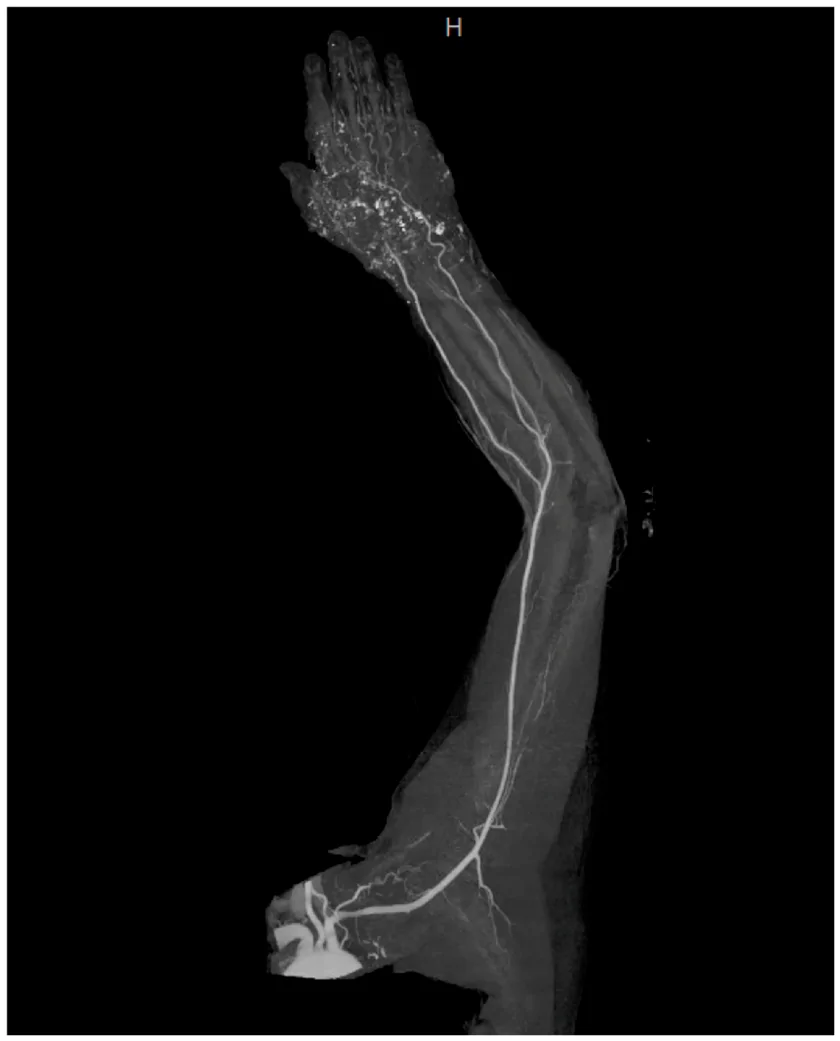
CT angiography of the left upper extremity following motor vehicle collision in Case 1. Three-dimensional angiographic reconstruction demonstrating distal radial artery injury at the level of the wrist. Associated CT findings included extensive carpal and carpometacarpal fracture–dislocations, including displaced comminuted fractures of the hamate and pisiform, dislocation of the trapezium and trapezoid, and fractures of the fourth and fifth metacarpal bases, with surrounding hematoma and surgical emphysema extending into the forearm.
